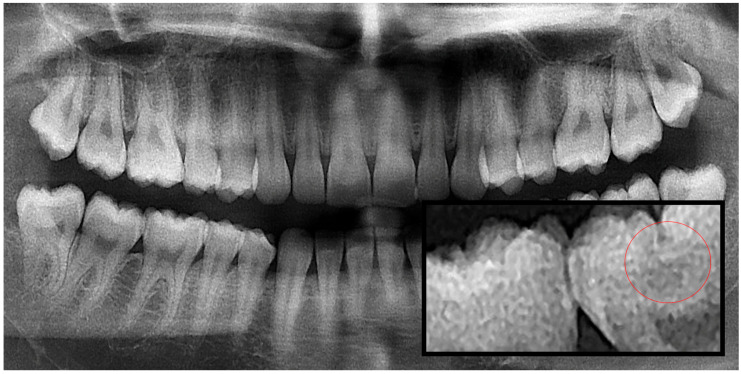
Panoramic radiography and segmented occlusal caries, red circle shows the caries.
